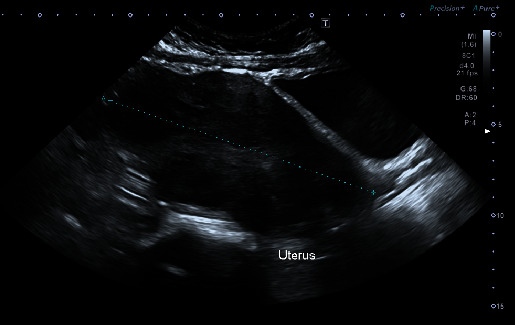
Transabdominal ultrasound of the patient: multiple intramural fibroids, thick poorly defined endometrium.
